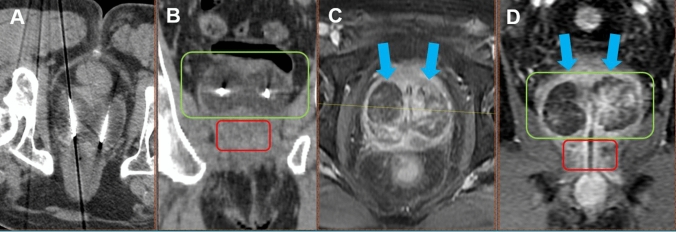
Intra- and post-procedural imaging of prostatic cryoablation. A Axial CT imaging demonstrates the converging course of the cryoablation probes in prone position in the right and left prostatic transition zone base. The image shows the positioning of the tip of the cryoprobes up to the ventral border of the prostate. B The coronal CT reformations confirmed the correct positioning of both probes at the same desired height over the base and middle transitional zone, avoiding the apex (to preserve the functionality of the external urethral sphincter). C Axial, T1-weighted, fat-suppressed contrast-enhanced MRI of the prostate 48 h after cryoablation. The converging ablation zones are well documented on both sides (arrows). Cryoablation remains limited to the prostate, with no extension outside the prostate capsule. D Coronal, T1-weighted, fat-suppressed contrast-enhanced MRI depicting converging cryoablation of the prostatic transitional zone base and middle third (arrows), with the deliberate omission of the prostate apex
